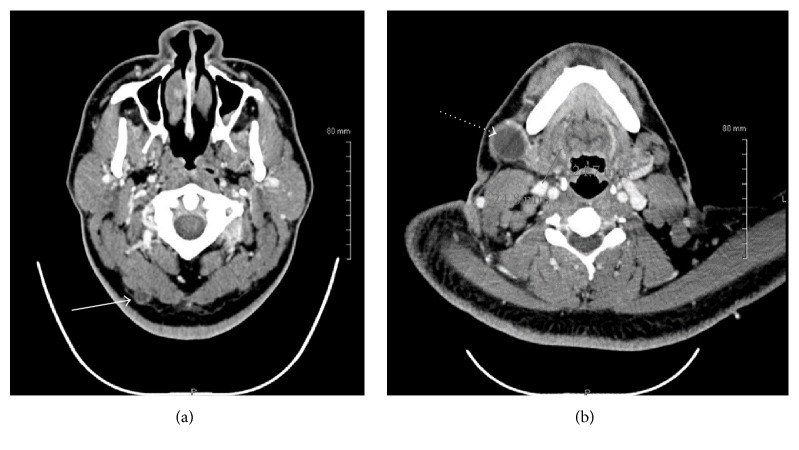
Computed Tomography (CT) scan of soft tissue neck demonstrating right occipital (solid arrow) and right jugulomandibular (dotted arrow) cervical lymphadenopathies.
